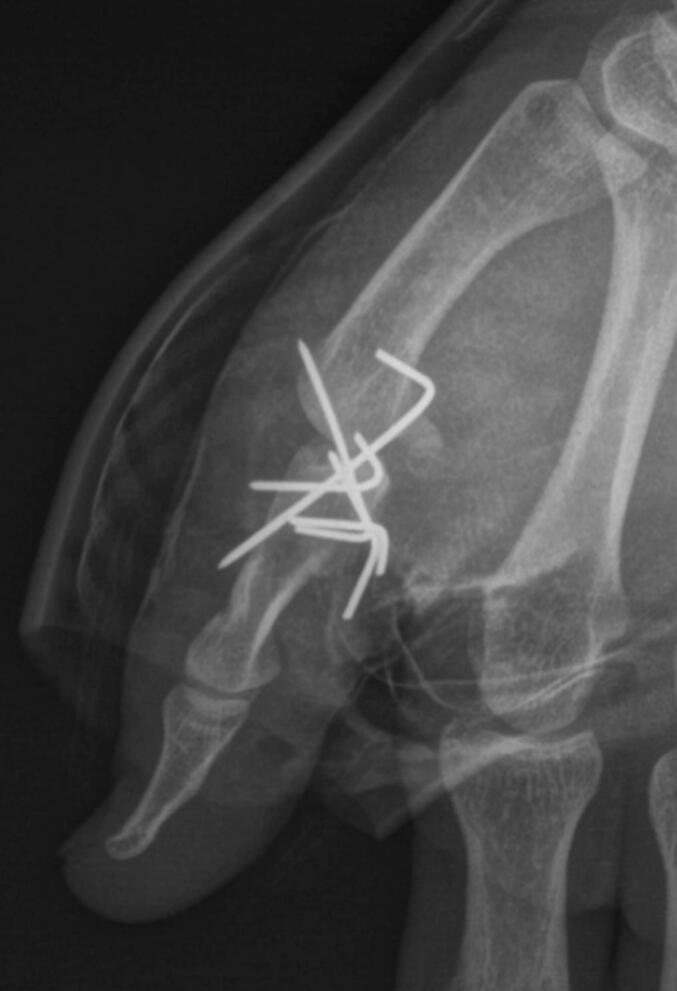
Lateral radiograph at the first operative stage during replantation.
